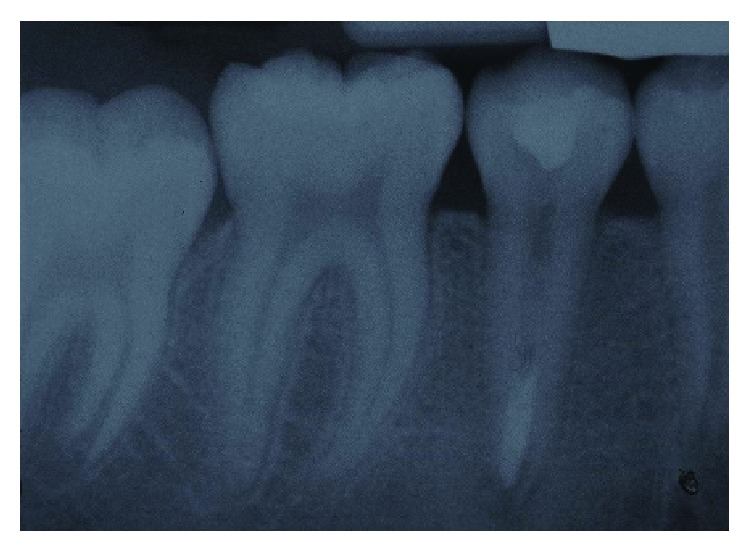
Periapical radiograph of tooth 45 after apexification with EndoSequence Root Repair Material.
